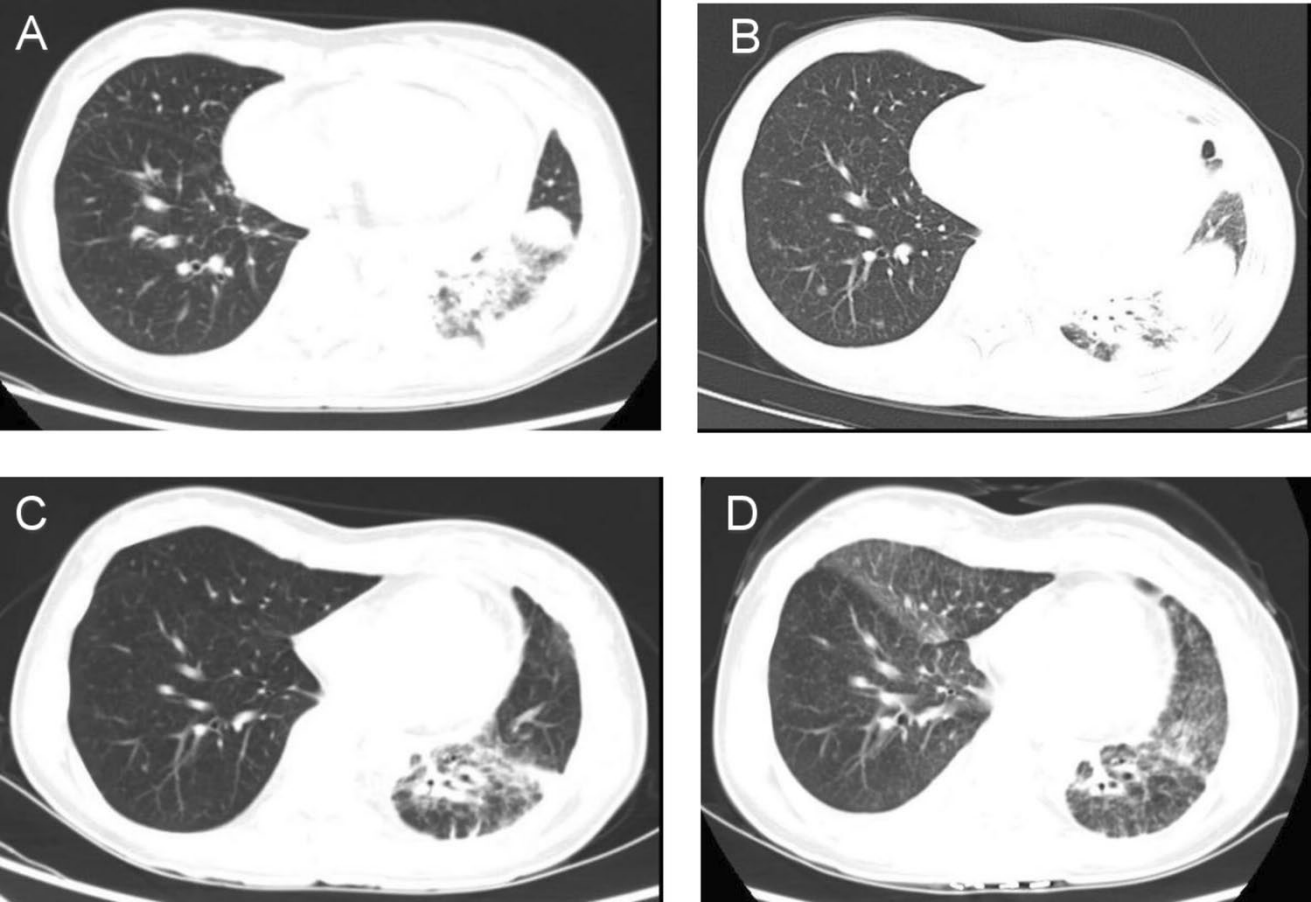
Chest CT scan results of the patient. A Before targeted therapy. B 8 months after targeted therapy: new nodules were found in both lungs. C 3 months after second-line treatment. D One year of second-line treatment. *CT* computed tomography
